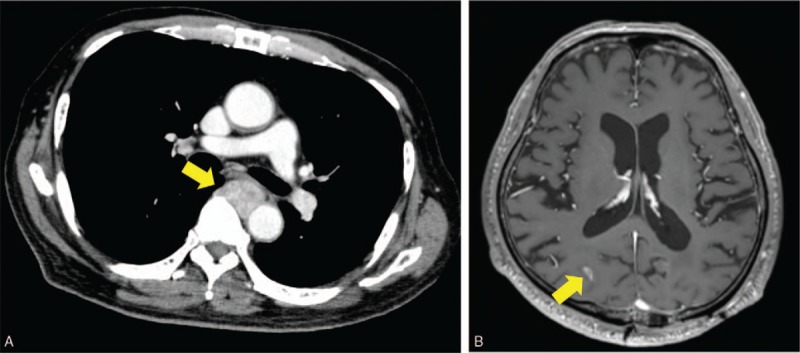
CT of the thorax and MRI of the head after 2 cycles of GD therapy. (A) CT showing lymph node metastasis around the artery (arrow). (B) MRI showing metastasis at the right occipital lobe (arrow). CT = computed tomography, GD = gemcitabine+docetaxel, MRI = magnetic resonance imaging.
